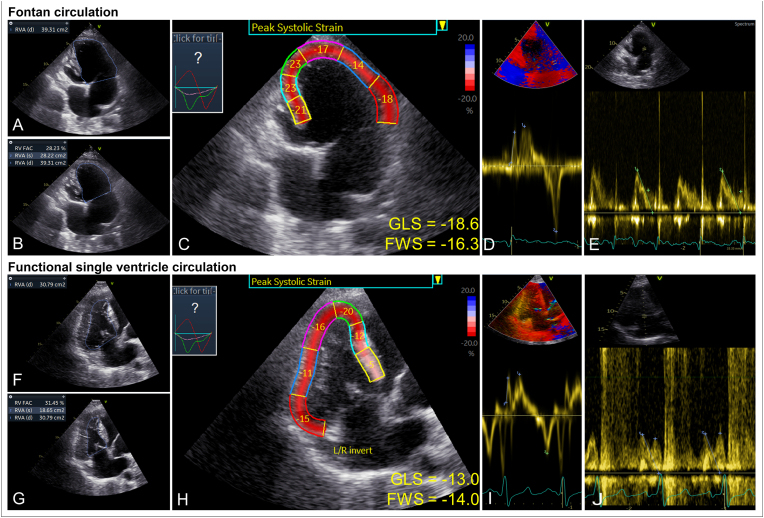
Title. TTE assessment methodology Fig. 1Caption. The top part of figure (A–E) shows the ventricular function assessment in a patient with tricuspid atresia and a left ventricle dominant Fontan circulation, the bottom part (F–J) in a patient with congenitally corrected transposition of the great arteries, double-outlet right ventricle, subaortic ventricular septal defect, and pulmonary atresia, palliated with a Glenn shunt and Blalock-Thomas-Taussig shunts. A&F) end-diastolic area (cm^2^), B&G) end-systolic area (cm^2^) and FAC calculation, C&H) strain analysis including GLS, segmental strain values, and calculation of the FWS by averaging the 3 lateral strain segments, D&I) tissue Doppler imaging measurements S’ (measurement 1, m/s), e’ (measurement 2, m/s), and IVA (slope measurement 3, m/s^2^), E&J) E and A velocities (m/s) measured on (at least) two complexes on pulsed-wave Doppler. *FAC, fractional area change; FWS, free wall strain; GLS, global longitudinal strain; IVA, isovolumic acceleration; RV, right ventricle; RVA, right ventricle area; TTE, transthoracic echocardiography.*
